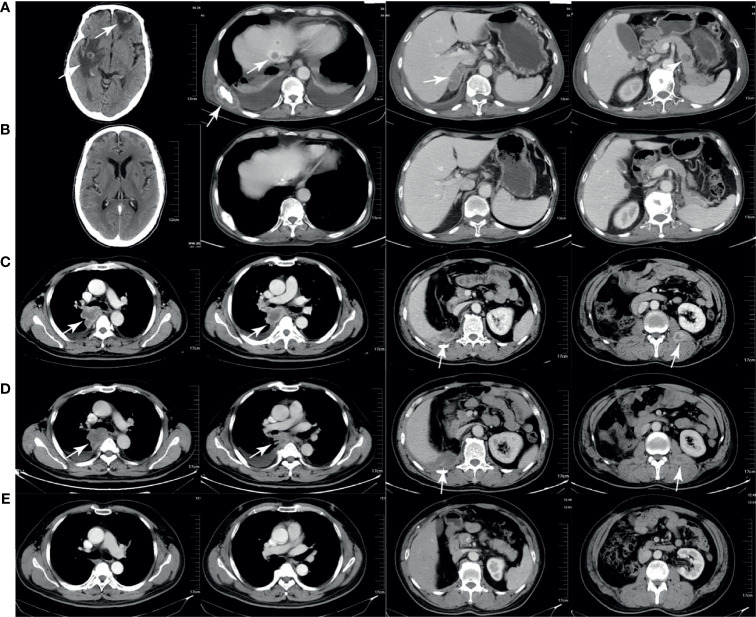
Computed tomography (CT) imaging of two patients with complete response. (A, B) CT scans of one patient before and after treatment. The brain, liver, bone, adrenal gland, and pancreas metastases (A) disappeared after treatment (B). (C–E) CT scans of another patient before treatment, during pseudoprogression, and after treatment. The lung, liver, and intramuscular metastases (C) enlarged after 2 months of therapy (D) and disappeared after 6 months of therapy (E).
